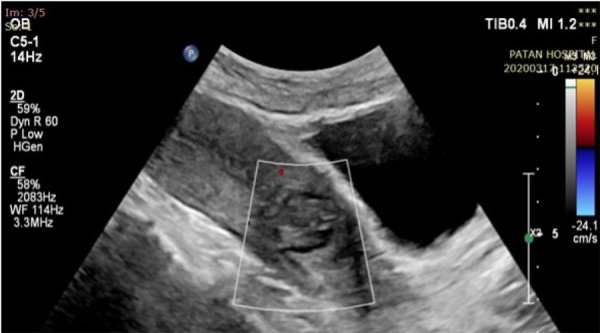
Post embolization Doppler ultrasonography shows the absence of turbulent vascularity of pseudoaneurysm.
